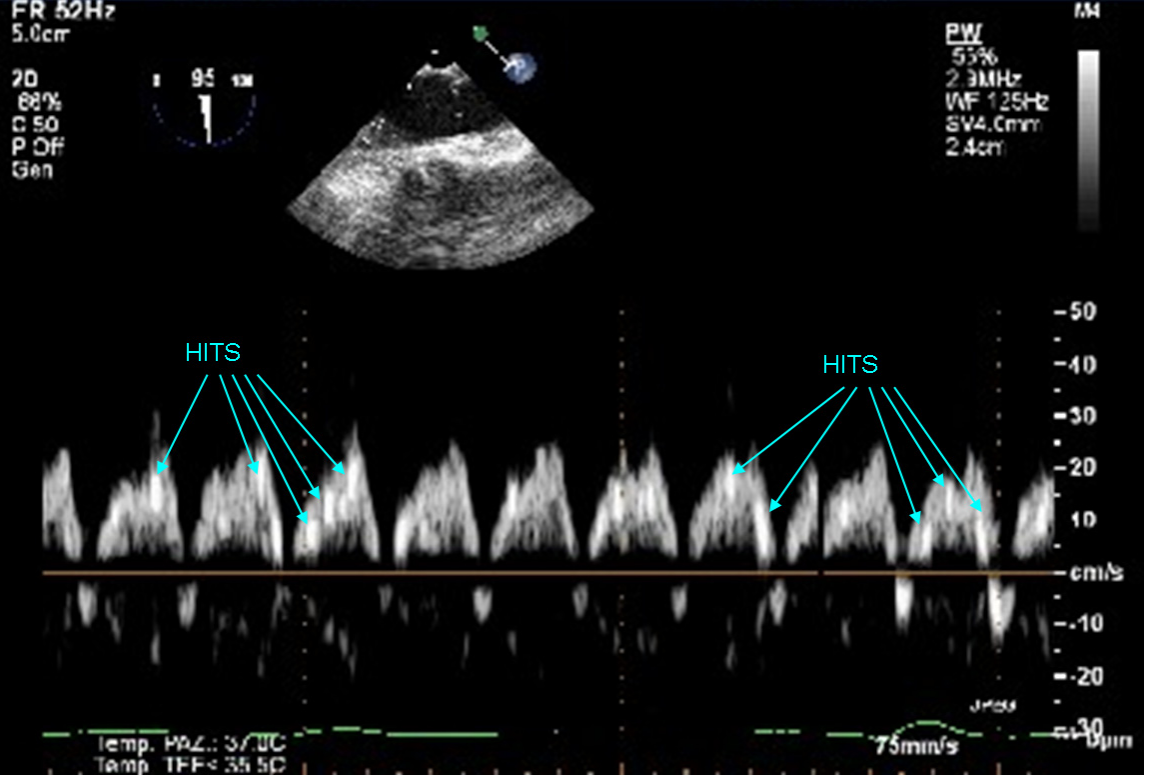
Two dimensional TEE Pulse Wave Doppler recording of HITS in the descending thoracic aorta long axis view during CPB.
